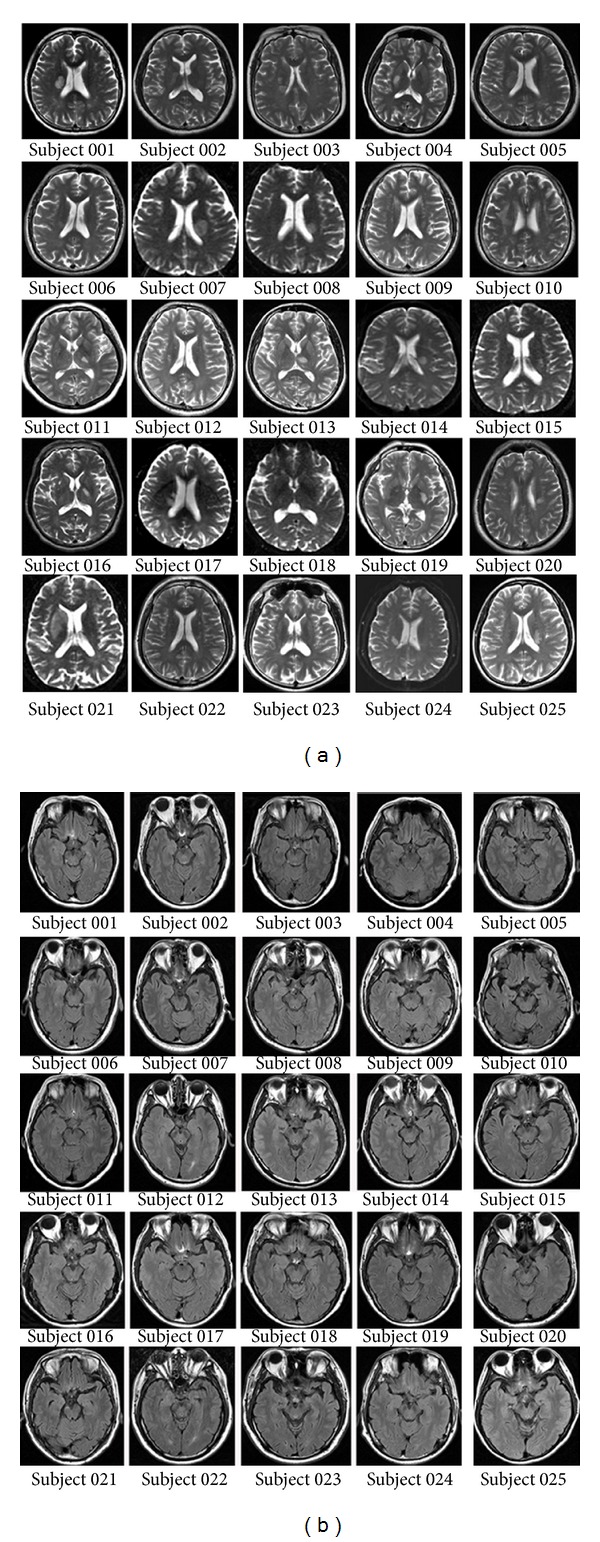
(a) Lesion locations of stroke patients on axial T2-weighted images acquired at the stroke onset when stroke patients were first enrolled in our hospital. (b) The morphology and signals of the brainstem of every stroke patient.
